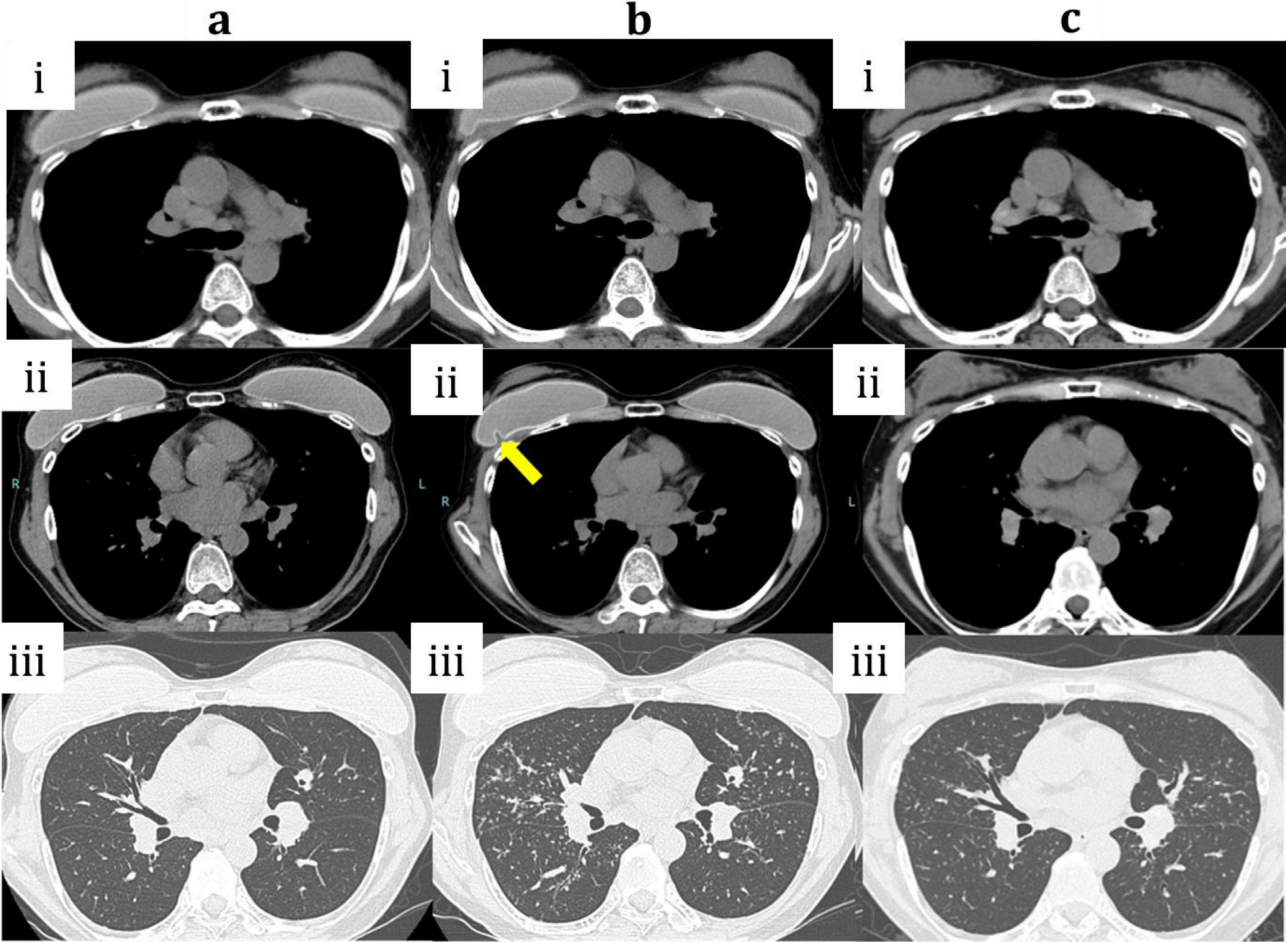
Chest CT findings. (a‐i) Mediastinal window: mediastinal and hilar lymphadenopathy is present. (a‐ii) bilateral subpectoral silicone implants are visible. (a‐iii) Lung window: subtle bilateral granular opacities are observed. (b‐i) Mediastinal window: mediastinal and hilar lymphadenopathy is slightly reduced in size compared with panel (a‐i), but remains enlarged. (b‐ii) deformation of the right silicone implant is evident (arrow). (b‐iii) Lung window: the granular opacities were distributed mainly along the bronchovascular bundles, consistent with a peribronchovascular pattern. (c‐i) Mediastinal window: mediastinal and hilar lymphadenopathy persists, with no significant change in size compared with panel (b‐i). (c‐ii) both implants have been removed. (c‐iii) Lung window: decreased density of bilateral granular opacities indicating improvement. CT, computed tomography.
